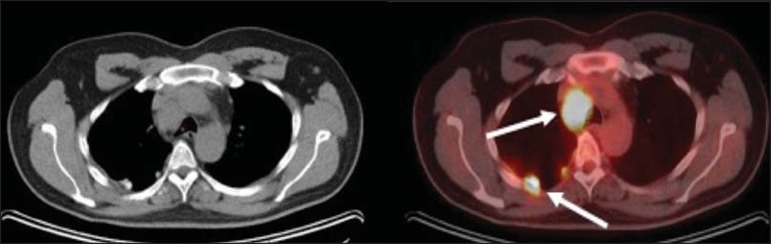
A 64-year-old woman submitted to PET/CT for the restaging of lung cancer.
